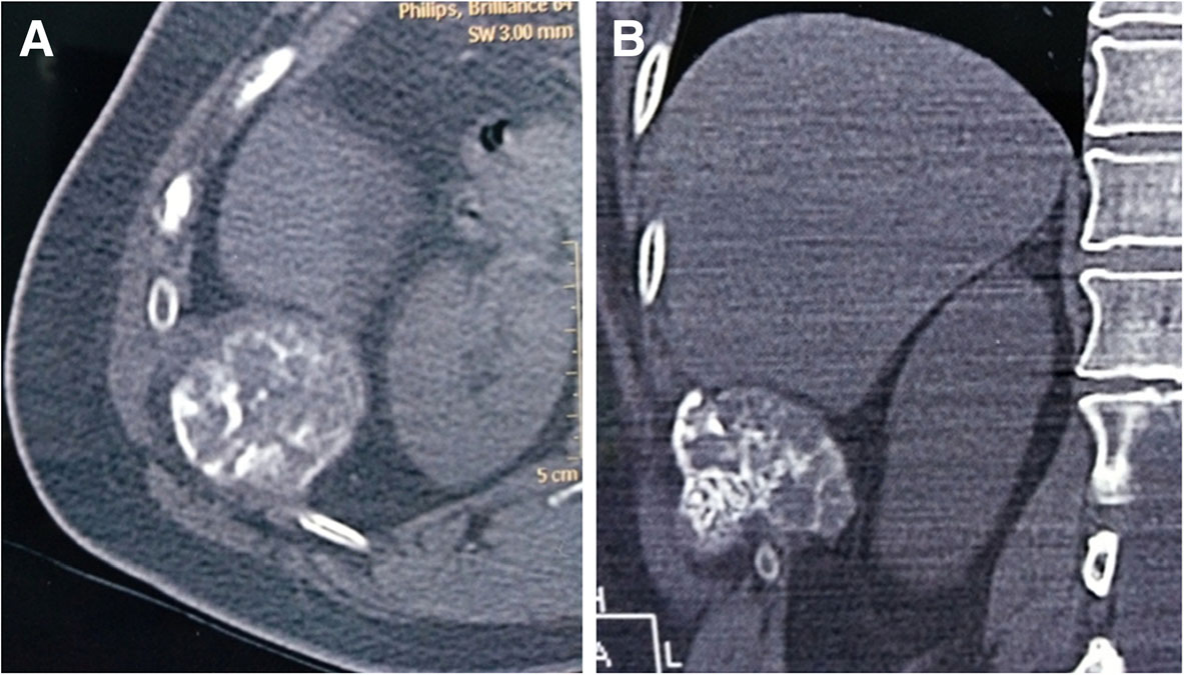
Chest computed tomography (CT), showing a tumor in the right eleventh rib(a), near the right lower lobe of liver (b), and an osteolytic eccentric expansive mass with sunburst calcification and focal cortical disruption
